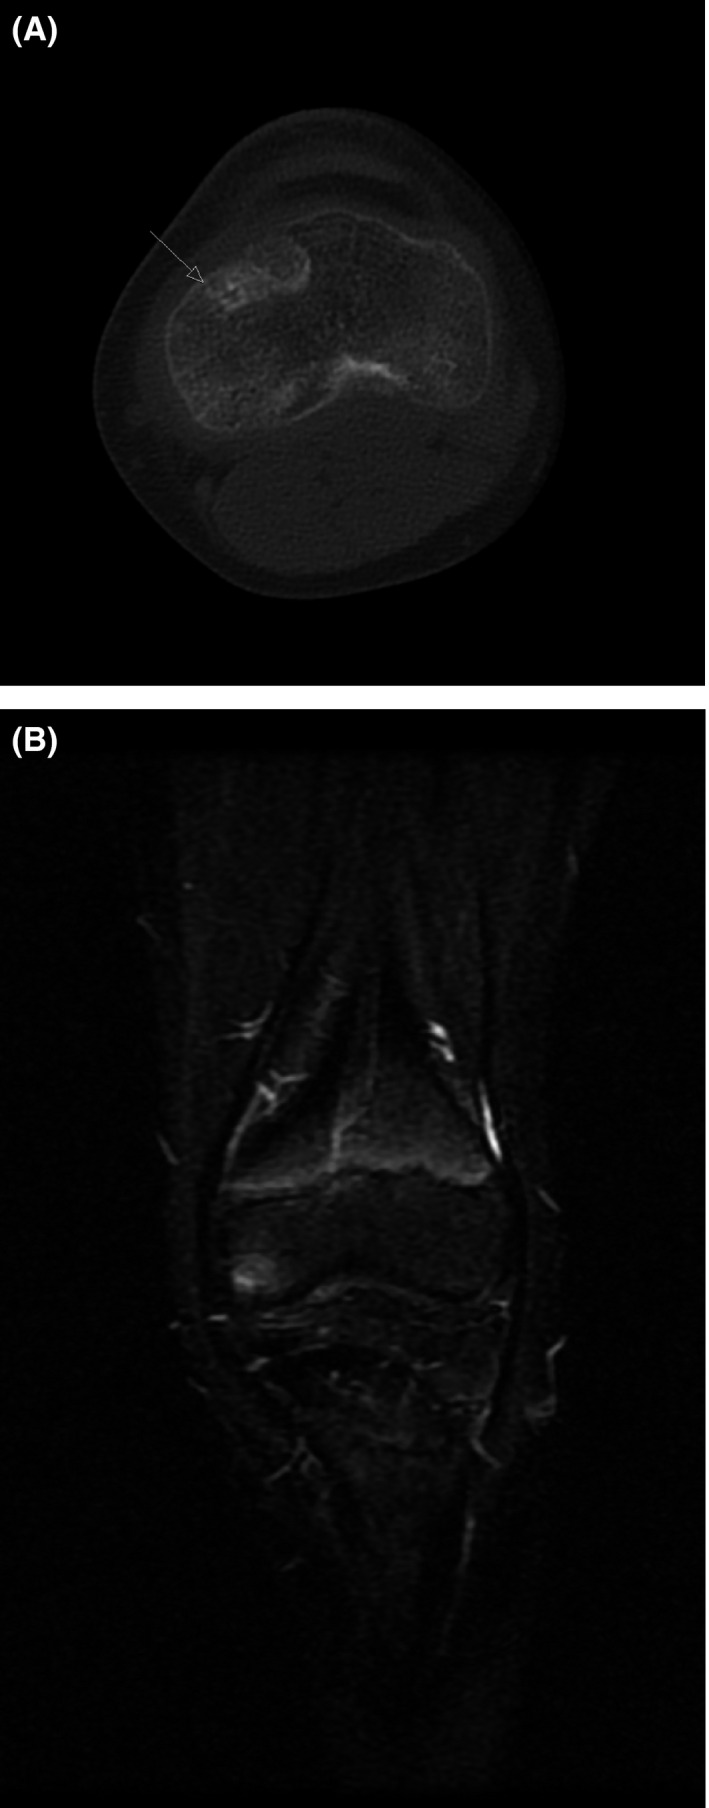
A‐B: Initial MRI and CT scan with an area of lucency with edema that led to incorrect diagnosis for a possible OO
